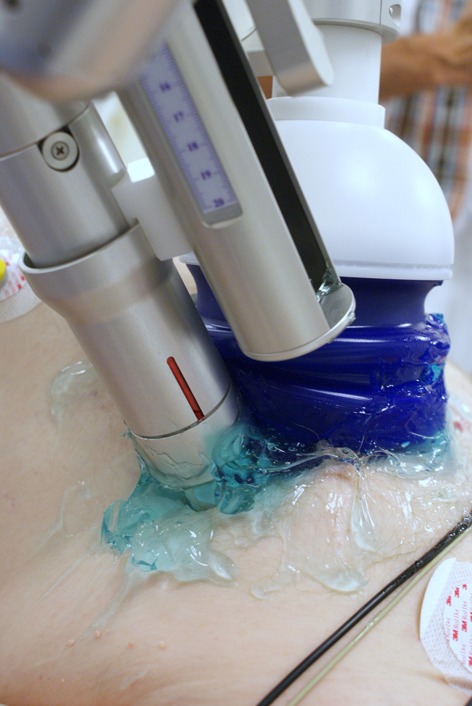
The shockwave applicator mounted in a holder together with a standard echocardiography transducer. Echocardiography is used to locate predefined ischaemic regions and to guide the focus of the shockwave applicator
